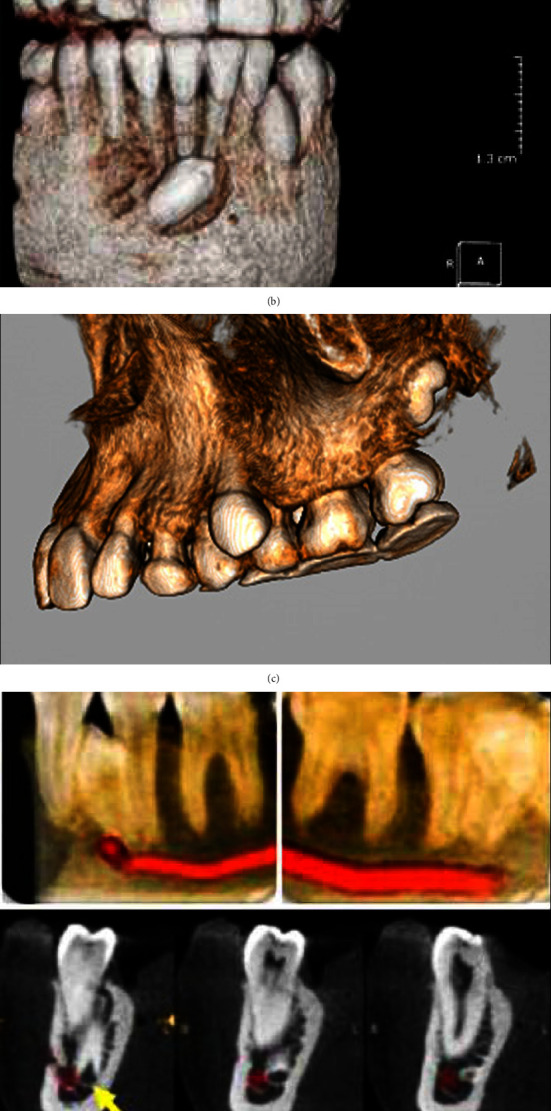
Mupparapu's classification using CBCT. (a) Type 1. (b) Type 2. (c) Type 3. (d) Type 4. (e) Type 5.
